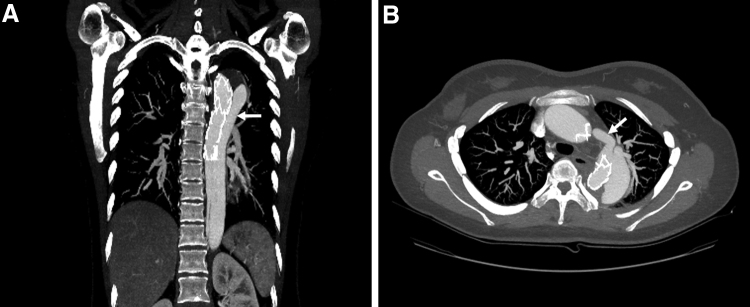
(A) Coronal CT performed 2 months after TEVAR showing a false lumen (arrow) filled with contrast agent, without inducing thrombosis. (B) Axial CT showing that the PDA (arrow) remains open. CT = computed tomography, PDA = patent ductus arteriosus, TEVAR = thoracic endovascular aortic repair.
